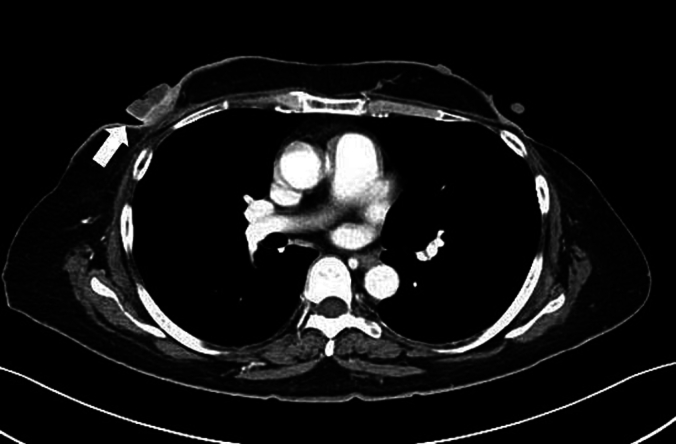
Enhanced CT; the right nipple is swollen and has a mass-like appearance compared to the opposite side. A slightly high-density area is observed directly below the nipple, but the shape is flat, the internal density is homogenous, and there are no signs of invasiveness around it, making it difficult to diagnose a recurrence based on this image alone.
